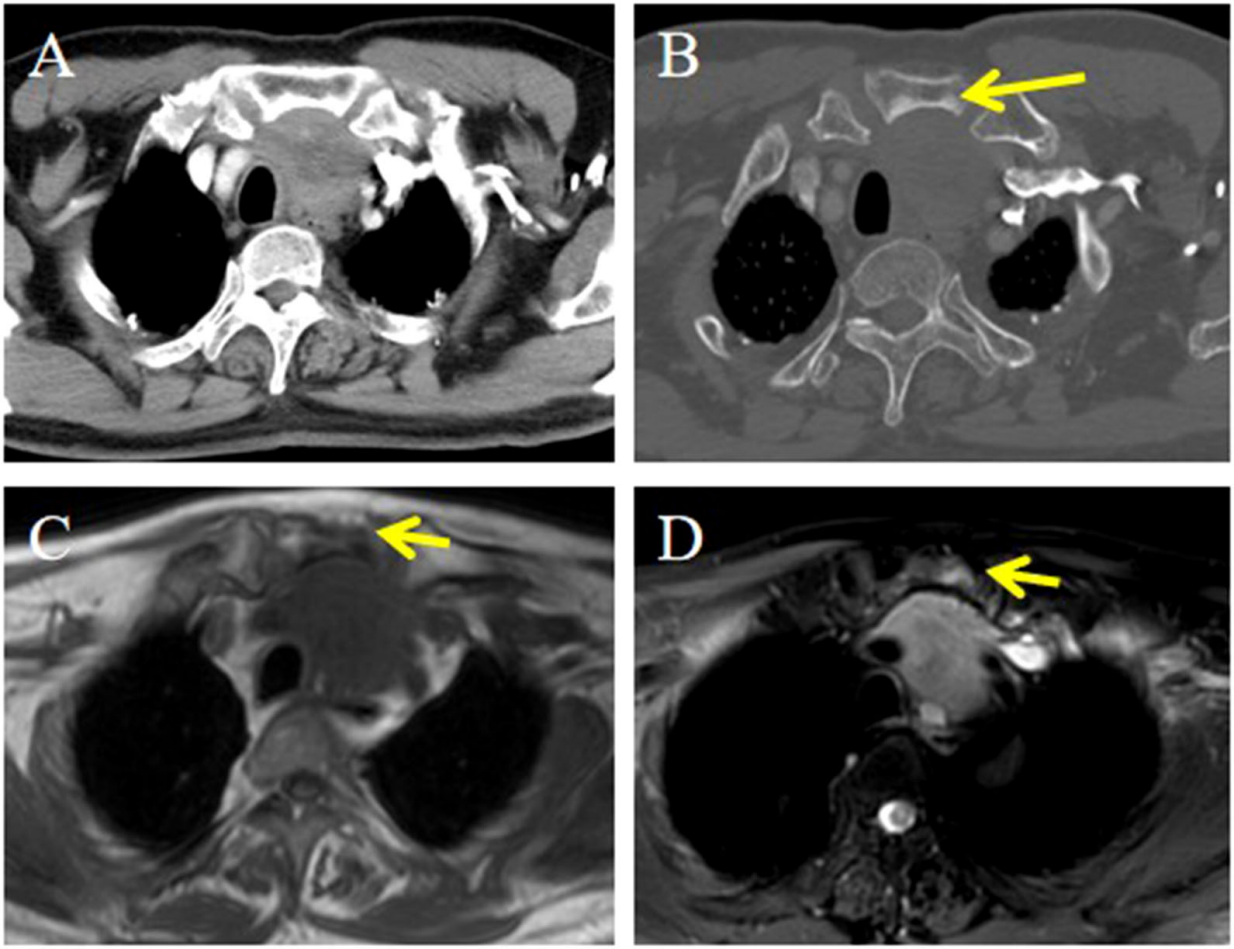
CT and MRI imaging of the ITTC. (A) Mass demonstrating heterogeneous enhancement at the left lower pole of the thyroid extending retrosternally into the upper mediastinum; (B) It showed invasion into the sternum, with heterogeneous density on CT (yellow arrow); (C, D) Invasive mass presented as hypointensity on axial T1-weighted imaging and slightly hyperintense on axial T2-weighted fat-suppressed sequence, invading to adjacent sternum resulting in abnormal bone signal (yellow arrow).
